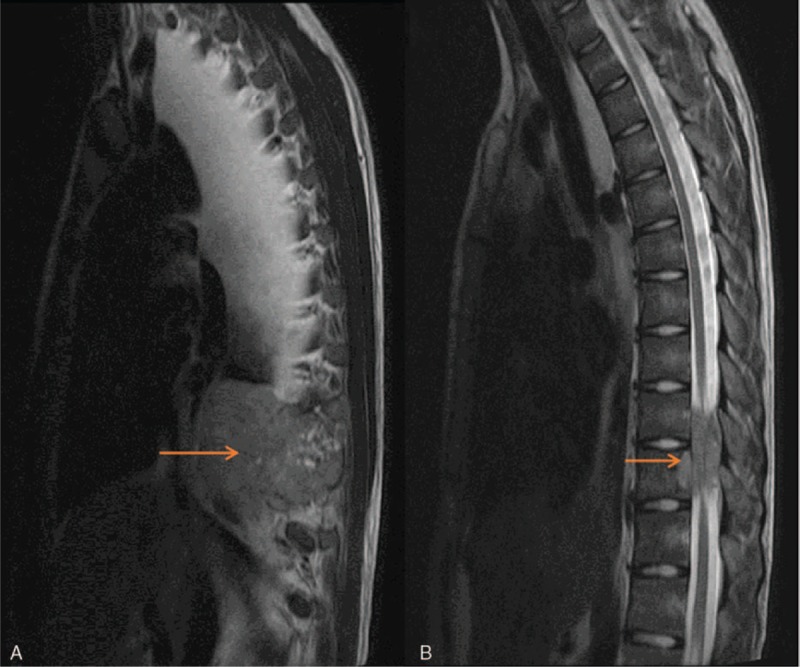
Preoperative sagittal MRI studies showing tumor completely violating the thecal sac and involving the adjacent vertebrae (A, B). MRI = magnetic resonance imaging.
